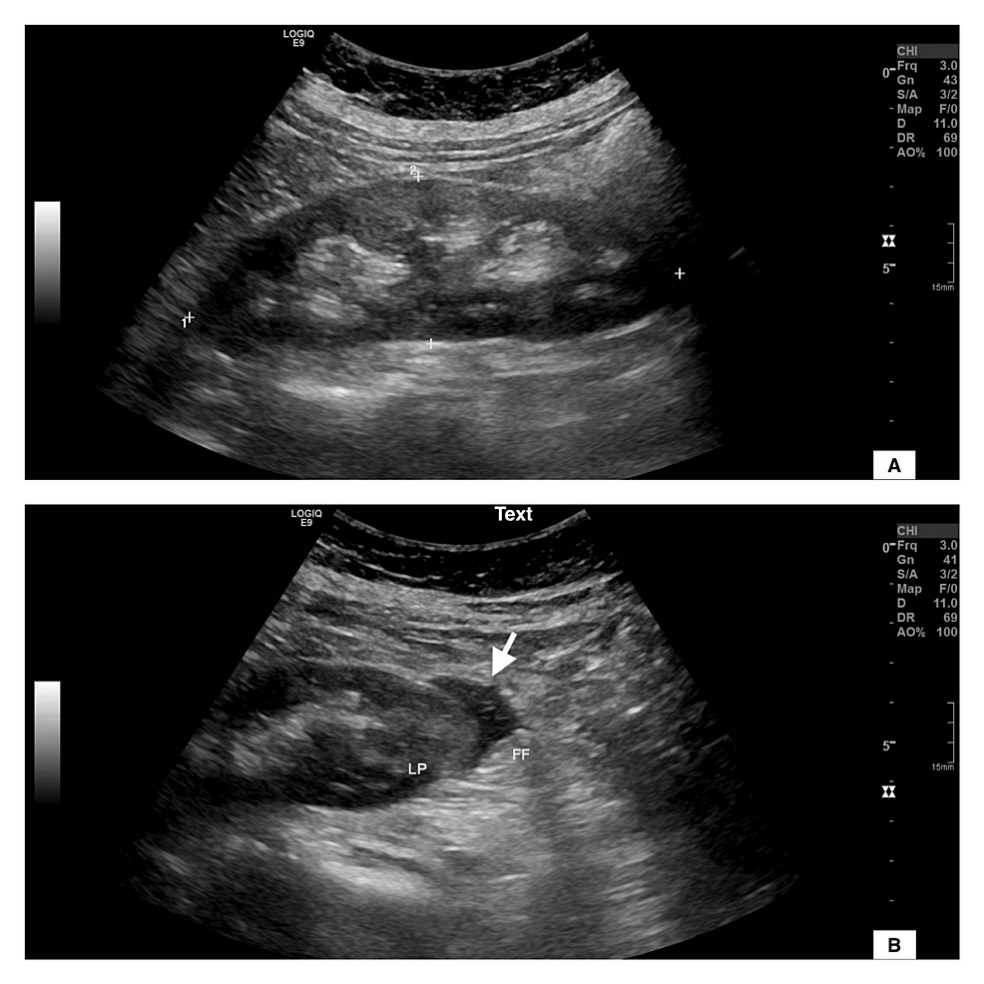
Greyscale ultrasound images in the longitudinal plane Seen is an unremarkable right kidney (A) apart from the minimal perinephric free fluid (white arrow in image B).
